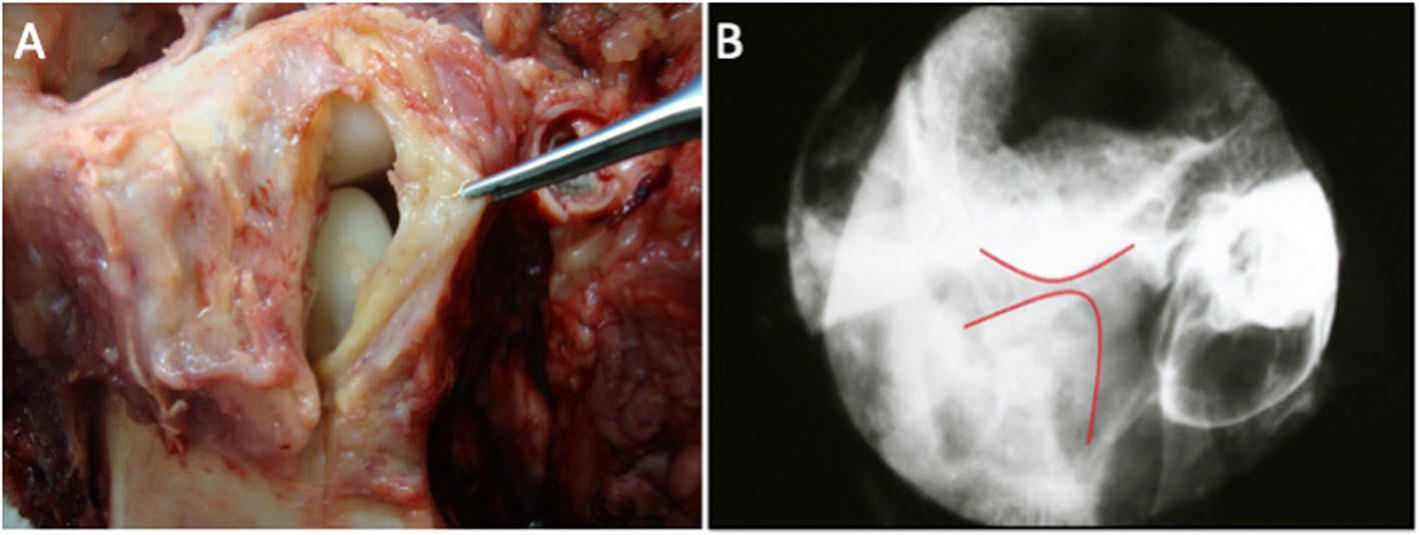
Macroscopic and imaging examinations in the postoperative period. A Post-mortem TMJ exposure and collection of peri-articular soft tissue samples. The image shows the clamping of the capsule, which covers the joint reconstruction for the evaluation of reconstruction stability; B Indication of the articular reconstruction site in the X-ray image
